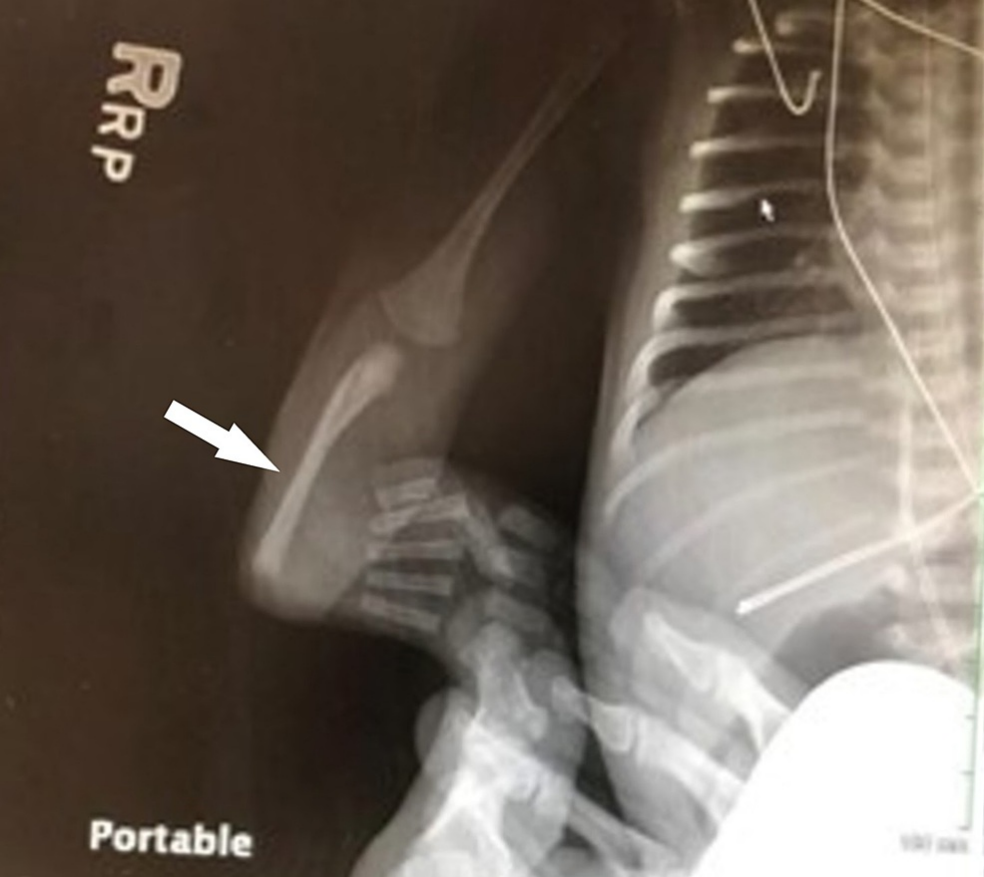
X-ray of the right arm demonstrating absent radius
